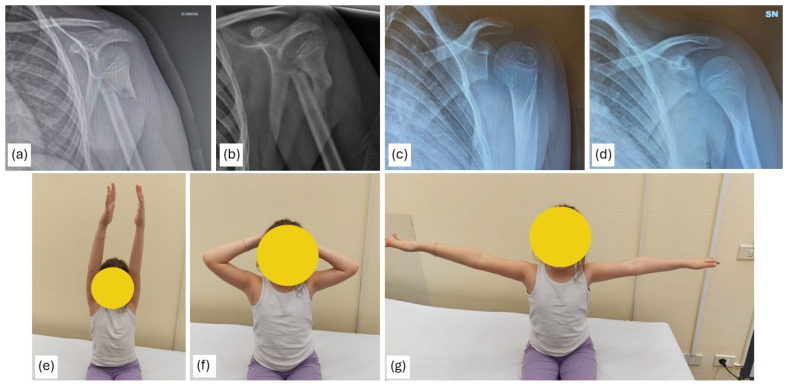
A 7-year-old patient with a Neer type IV fracture treated conservatively. Follow-up radiographs at 1 month (a,b) and at 10 months are shown (c,d). Clinical follow-up at 10 months demonstrated complete restoration of joint range of motion (e–g).
